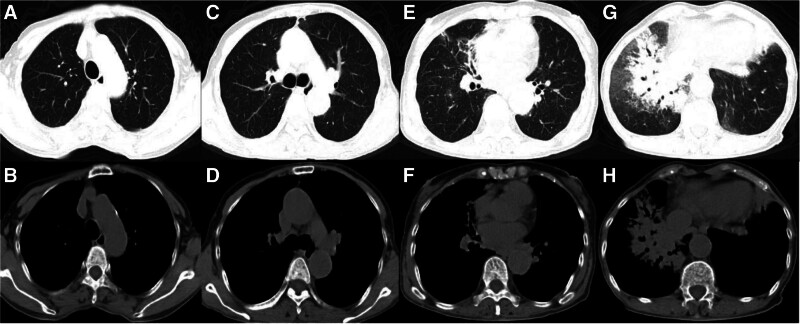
2021-05-25 chest CT: (A–H) show slight dilation of the bronchus in the middle lobe of the right lung, the upper lobe of the left lung, and the lower lobe of both lungs, and the shadow of the surrounding spots, significant in the middle and lower lobe of the right lung, partial lung tissue consolidation, visible air bronchus, fuzzy nodules in both lungs, calcified nodules in the right subpleura, and calcification in the aorta and coronary walls. CT = computed tomography.
